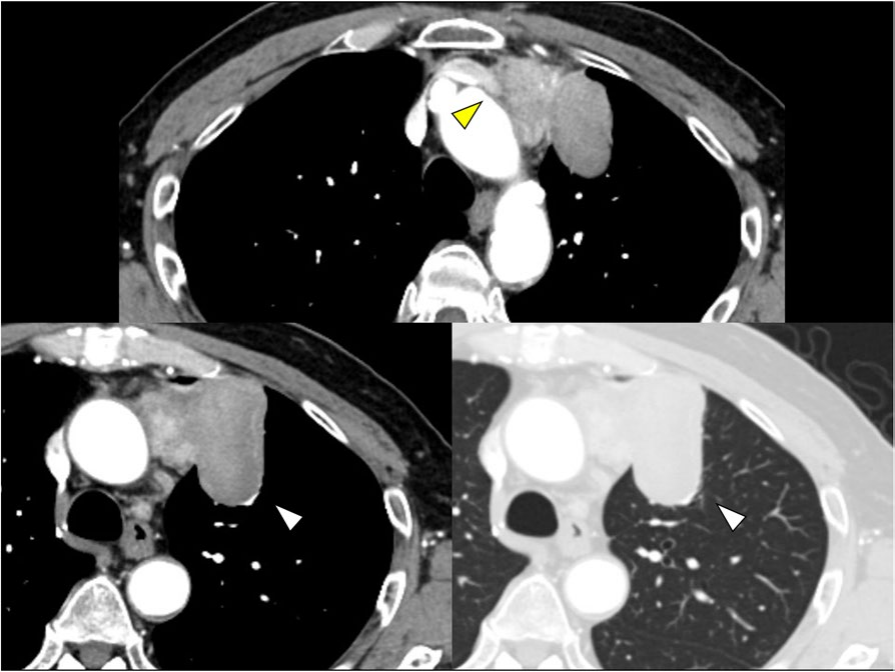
Chest computed tomography revealed a 7.1-cm tumor in the left anterior mediastinum with suspected involvement of the left brachiocephalic vein (yellow arrowhead) and upper lobe of the left lung (white arrowheads)
